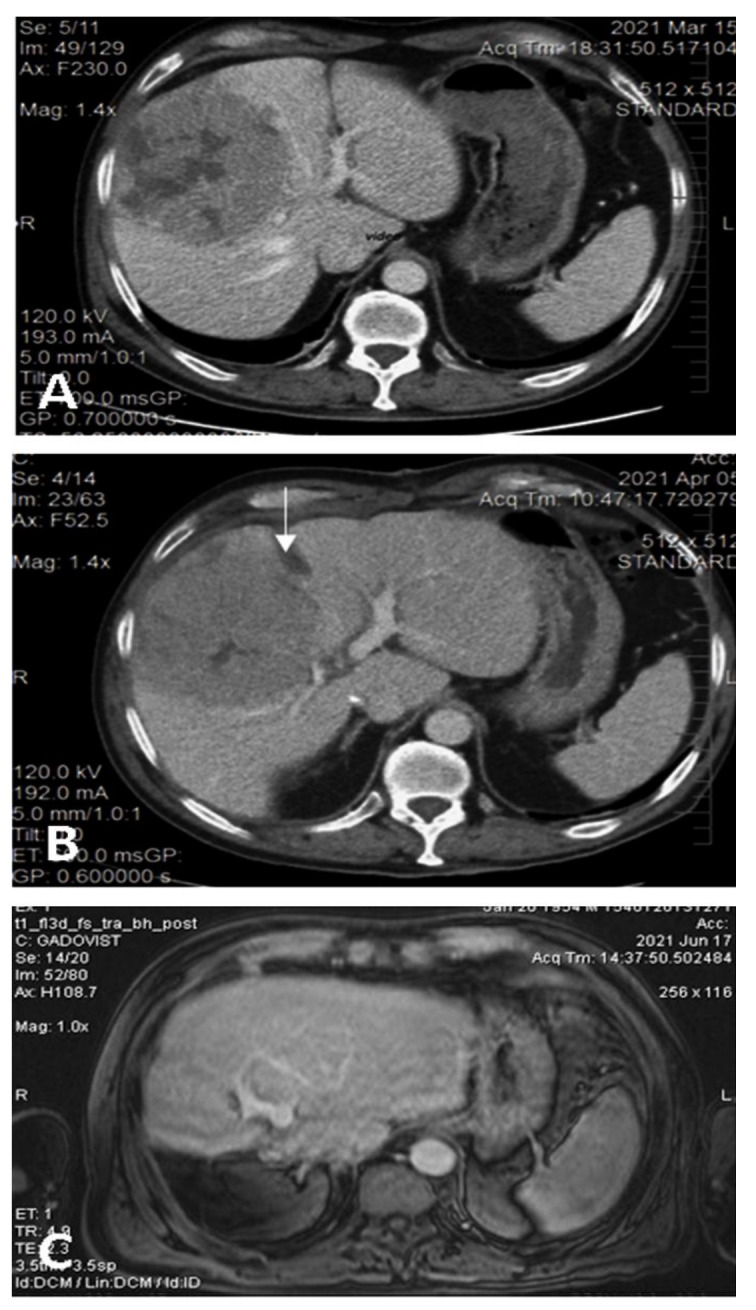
(A). Preoperative CT showing the large HCC located in segments 5 and 8 with extension to segments 6, 7, 4, compressing the middle hepatic vein; volumetry: volume of segments 2 and 3 16.8% of total functional liver volume, volume of FLR 27.8%. (B) Interstage CT showing the liver partitioning, absence of contrast in the right portal vein (due to ligation), and sufficient growth of FLR (38.5% of total functional liver volume). (C) Postoperative CT with well-perfused, non-dilated bile ducts, and tumor-free remnant liver.
